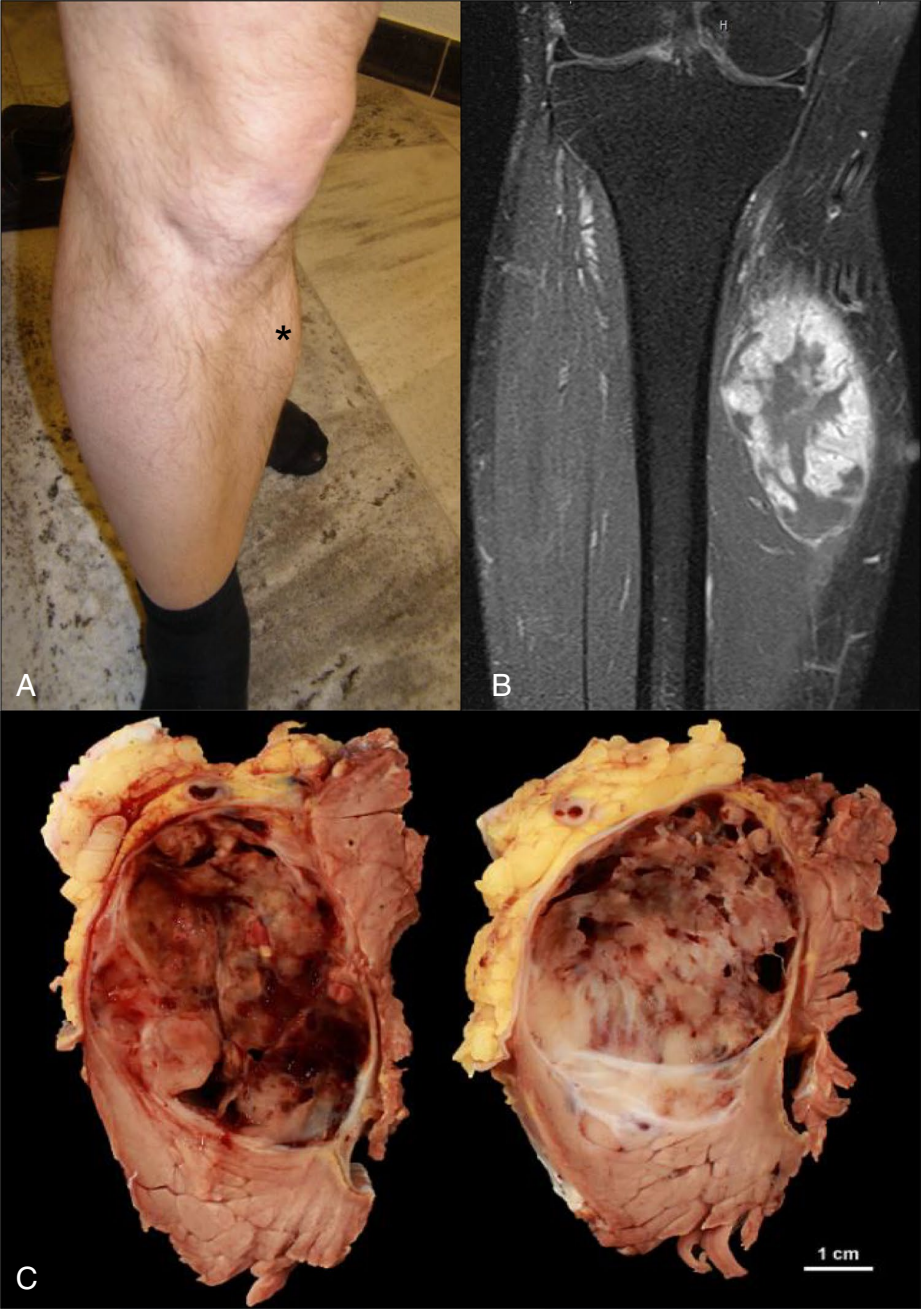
Presentation of the tumor. Clinical presentation of the tumor in form of a medial swelling (A, asterisk). MRI exam in T1 sequence and coronal plain showing no infiltration of the tibia (B). Macroscopic presentation of the resected tumor (C)
